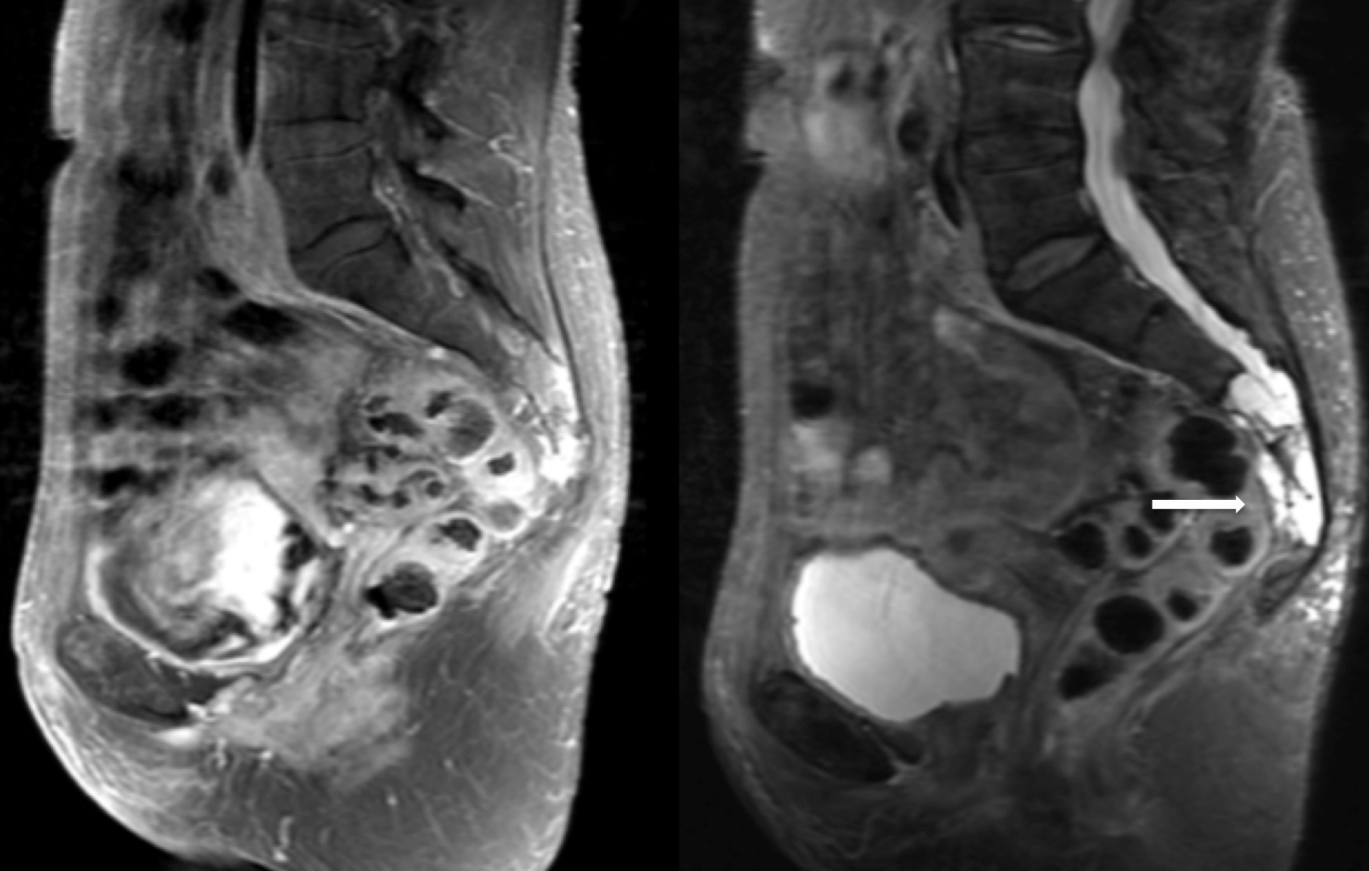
Patient 2 - Imaging findings MRI demonstrates a 4 x 2 x 4 cm sacral lesion involving the S2, S3, S4, and S5 nerve roots.
